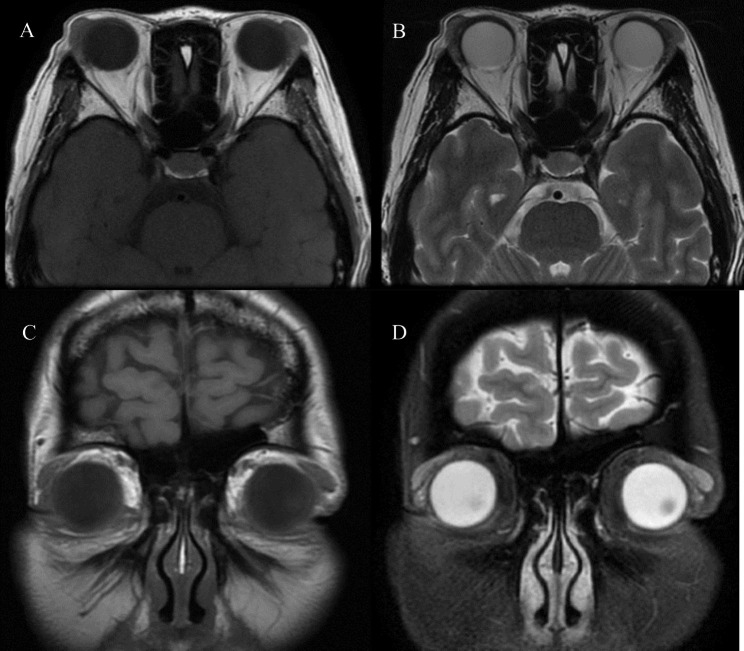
MR images of the orbit. A. T1-weighted images (T1WIs) of the orbital transection showing bilateral lacrimal glands moved forward and no abnormalities in the eyelids, extraocular muscles, nerves, or other tissues. B. Transverse orbital T2WIs showing bilateral lacrimal glands moved forward. C. Coronal T1WIs of the orbits showing bilateral lacrimal glands prolapse. D. Enhanced orbital scan showing bilateral lacrimal glands prolapse
